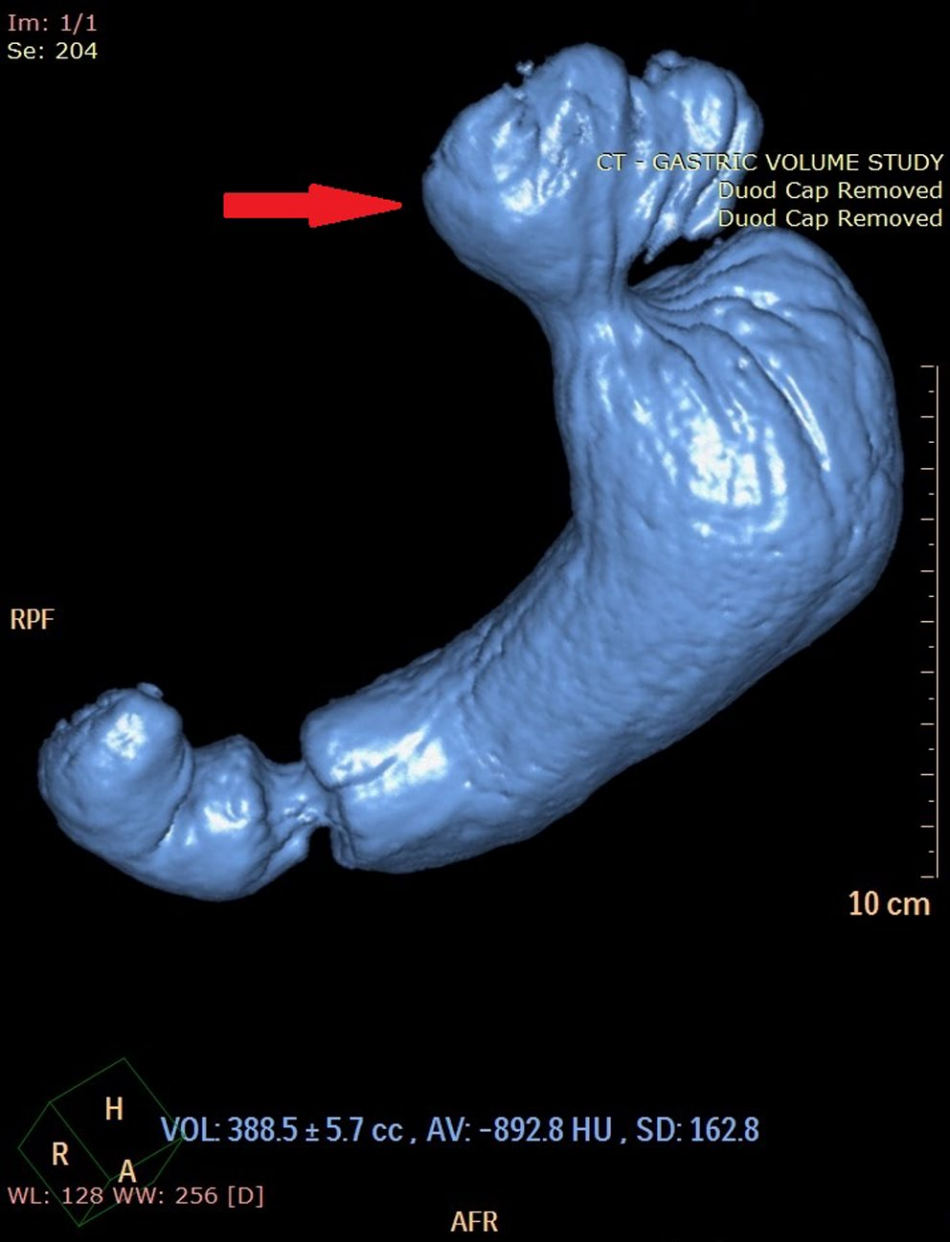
Preoperative investigation of patient #1 (76-year-old female with reflux symptoms) with a coronal image of CT (left) and 3D volumetric rendering of the same Fizz-CT (right) showing a primary hiatus hernia (red arrow)
